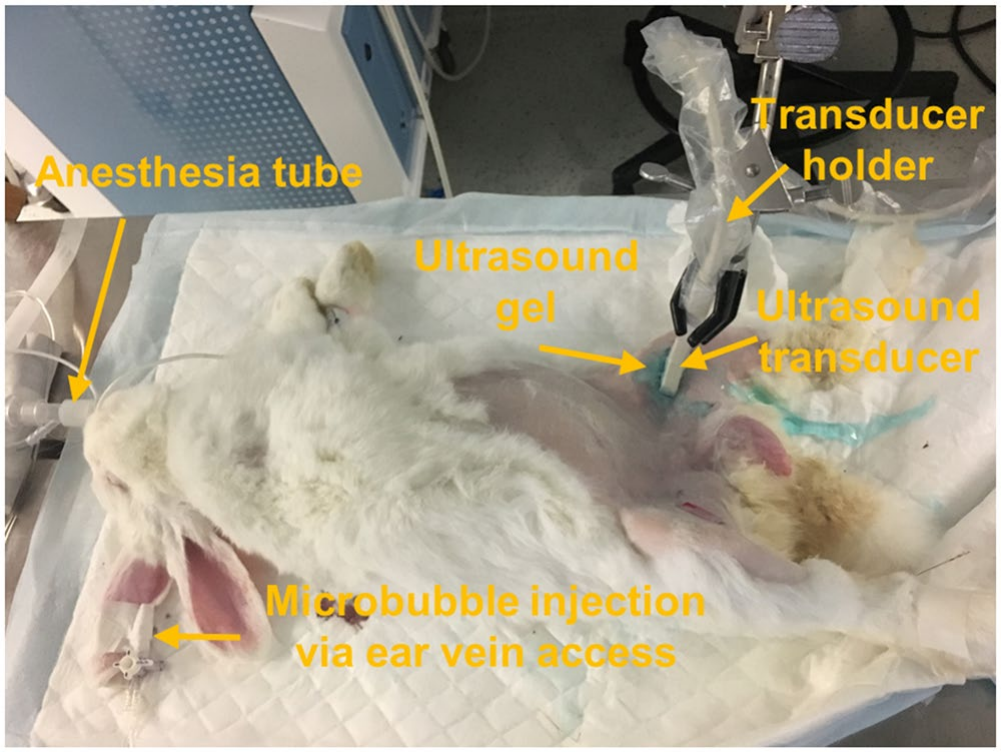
Experiment setup of rabbit imaging. Microbubbles were injected via ear vein access. A hockey stick linear array ultrasound transducer was used for imaging. Transducer holder is used to removing operator-dependent motion in this study.
